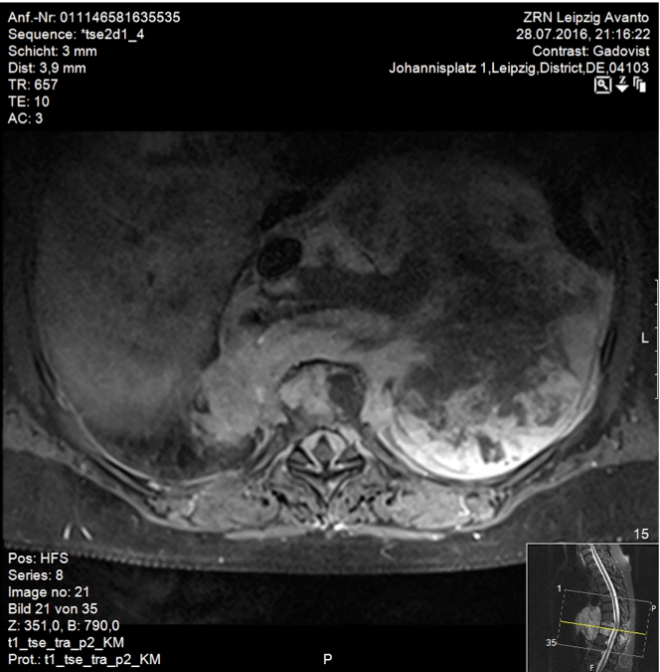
T1-weighted, contrast enhanced transverse MR image of the thoracic spine. Inhomogeneous gadolinium distribution. Solid mass in the posterior inferior mediastinum with spinal canal infiltration and myelon compression.
